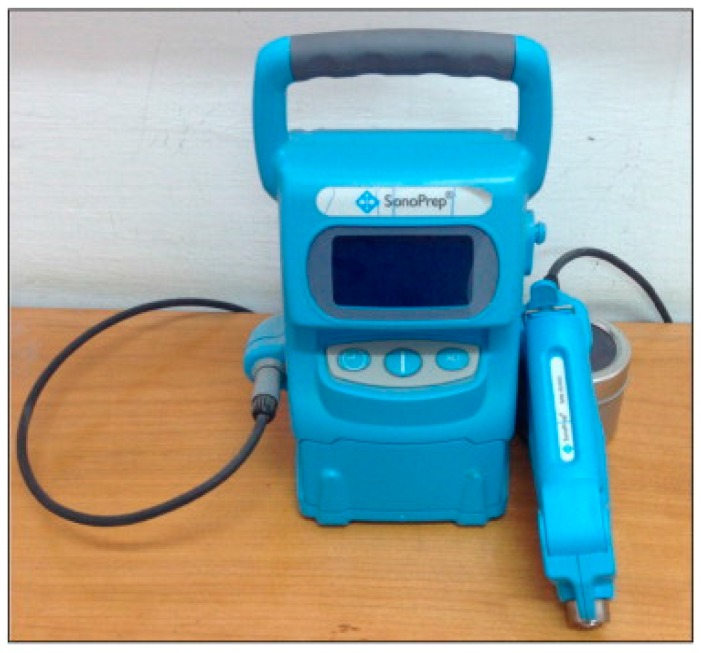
The SonoPrep^®^ ultrasound device (Reprinted from [50] with permission. Copyright 2014 Elsevier).
